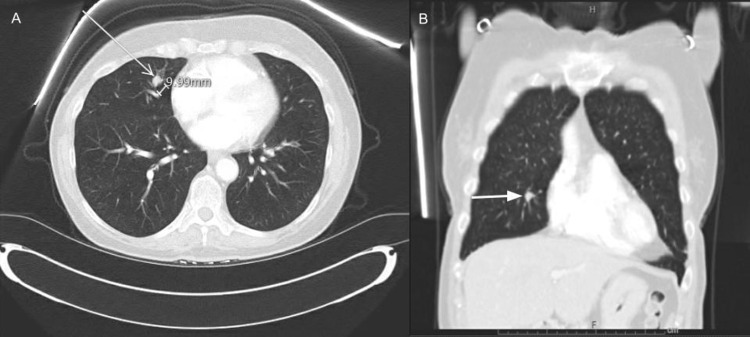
CT scan of the chest showed a right middle lobe pulmonary nodule approximately 9.99 mm in diameter concerning for malignancy (A: axial view, B: coronal view).
